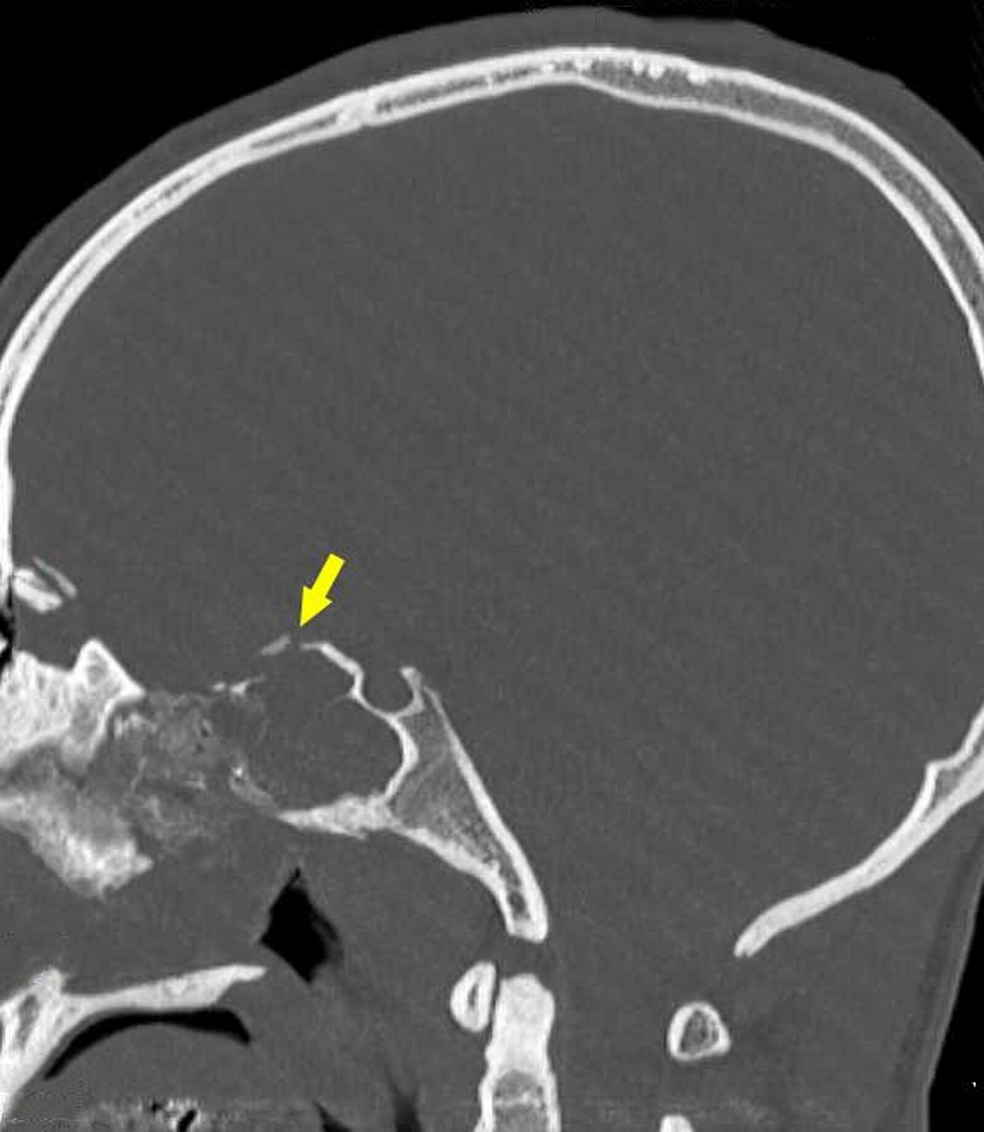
Sagittal CT image showing fracture of the roof of the sphenoid sinus. Arrow shows discontinuity and displacement of bone at the roof of sphenoid sinus indicating fracture of the roof of sphenoid sinus. CT: computed tomography.
